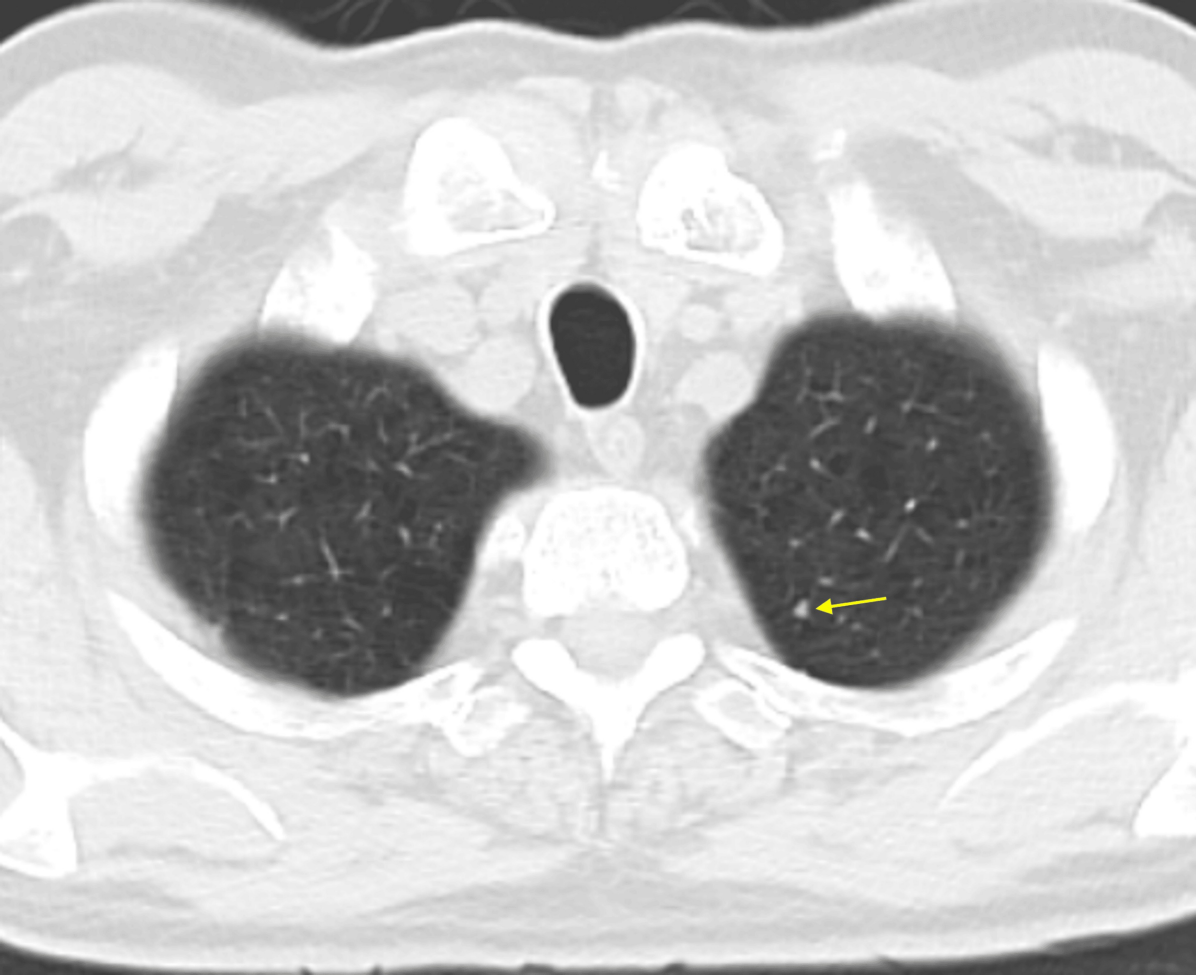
Chest CT scan in October 2022 showing a small 3-4 mm nodule in the upper lobe of the left lung CT: computed tomography
